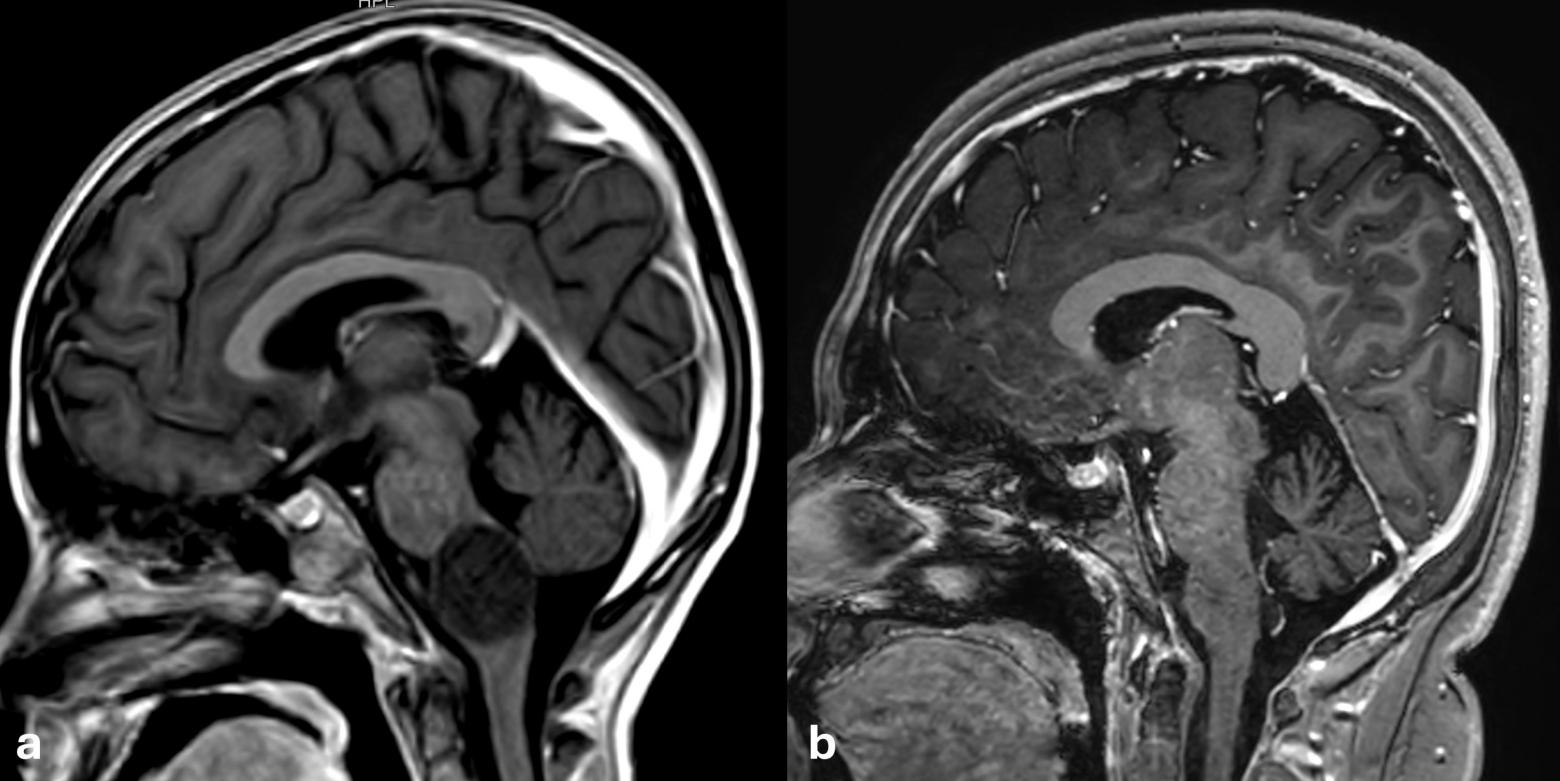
Sagittal T1-weighted MR images after contrast administration at the ages of 8 years (a) and the current age of 15 years (b) in Patient 1. The patient presented with a focal expansive tumor in the medulla oblongata, which significantly decreased in volume and recovered normal signal on T1 and T2 sequences (not shown). The lesion did not enhance after intravenous gadolinium administration.
